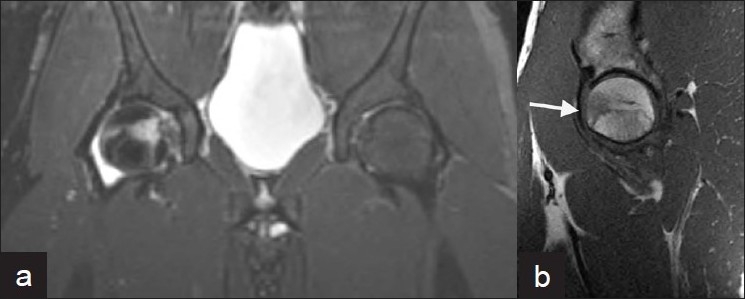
MRI six weeks after injury. The coronal fast spin echo T2- weighted image with fat saturation (a) and the sagittal T1-weighted (b) image show a new abnormality in the anterior aspect of the right femoral head, which is with low signal intensity on T1 weighted and high signal intensity on T2 weighted. (Images obtained on a 1.5T General Electric MR scanner)
